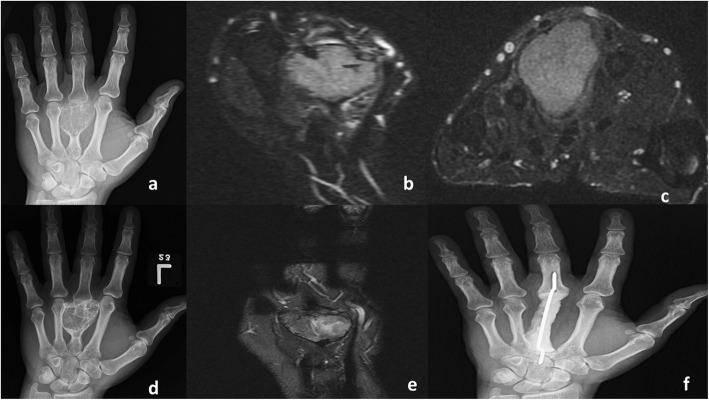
A 39-year-old male who was treated by denosumab for approximately 7 weeks then underwent en bloc surgical excision and non-biological reconstruction by cement/K-wire. Plain radiograph (a) and MRI (b) before treatment shows expansile metacarpal lytic lesion with corresponding high FS T2WI internal signal, and after treatment demonstrates marginal sclerosis and internal ossification on the X-ray (d) and newly developed areas of intermediate signal on follow-up MRI (e). Post-operative AP radiograph (f)
